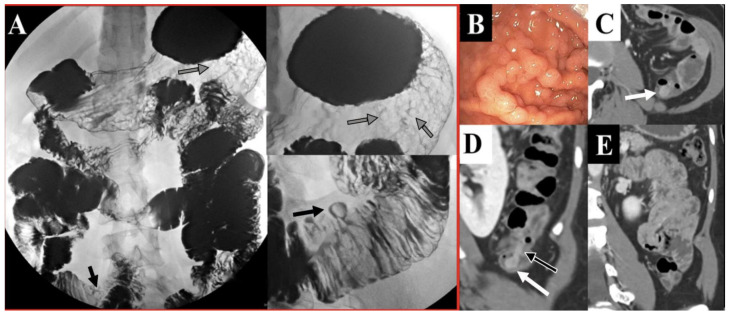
A 23-year-old female with known PJS. (Panel A) Upper GI series with barium contrast. Multiple filling defects throughout the stomach (gray arrows, upper figure) and jejunum (black arrows, lower figure) are characteristic of PJS. Presence of these polyps was confirmed by upper endoscopy (Panel B). (Panels C and D) Contrast-enhanced CT scan with enhancing polypoid lesions (white arrows) in the descending colon (axial section in panel C, coronal images in panels D and E). One of the polyps appears to have a thin stalk (black arrow with white border).
